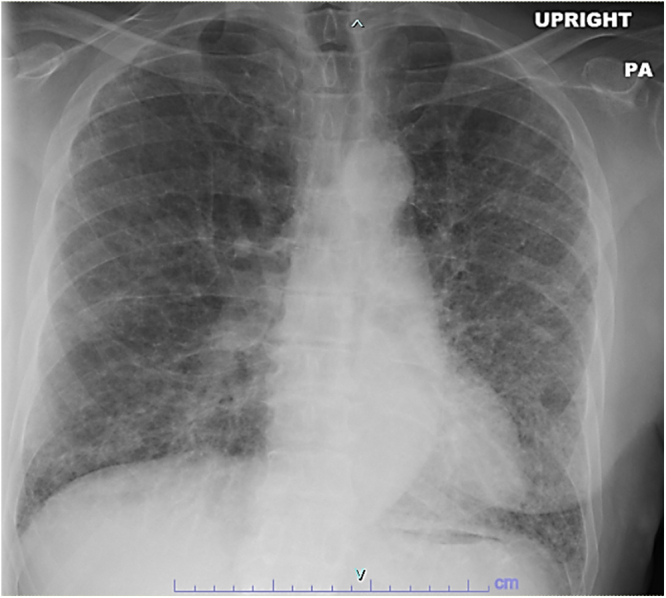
Initial chest X-ray showing diffuse bilateral reticular opacities.
